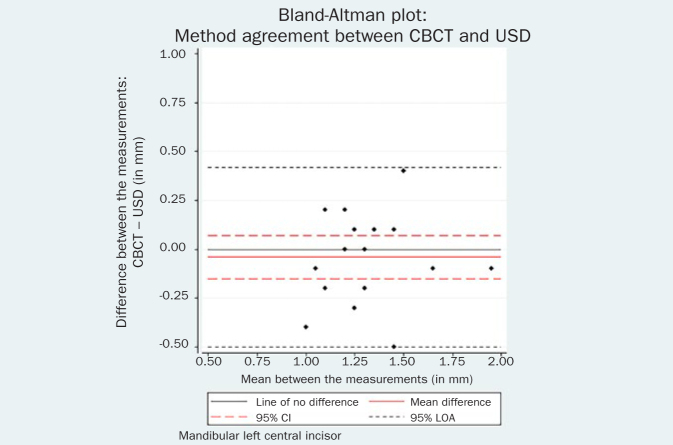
Bland-Altman plot CBCT vs USD 31.
